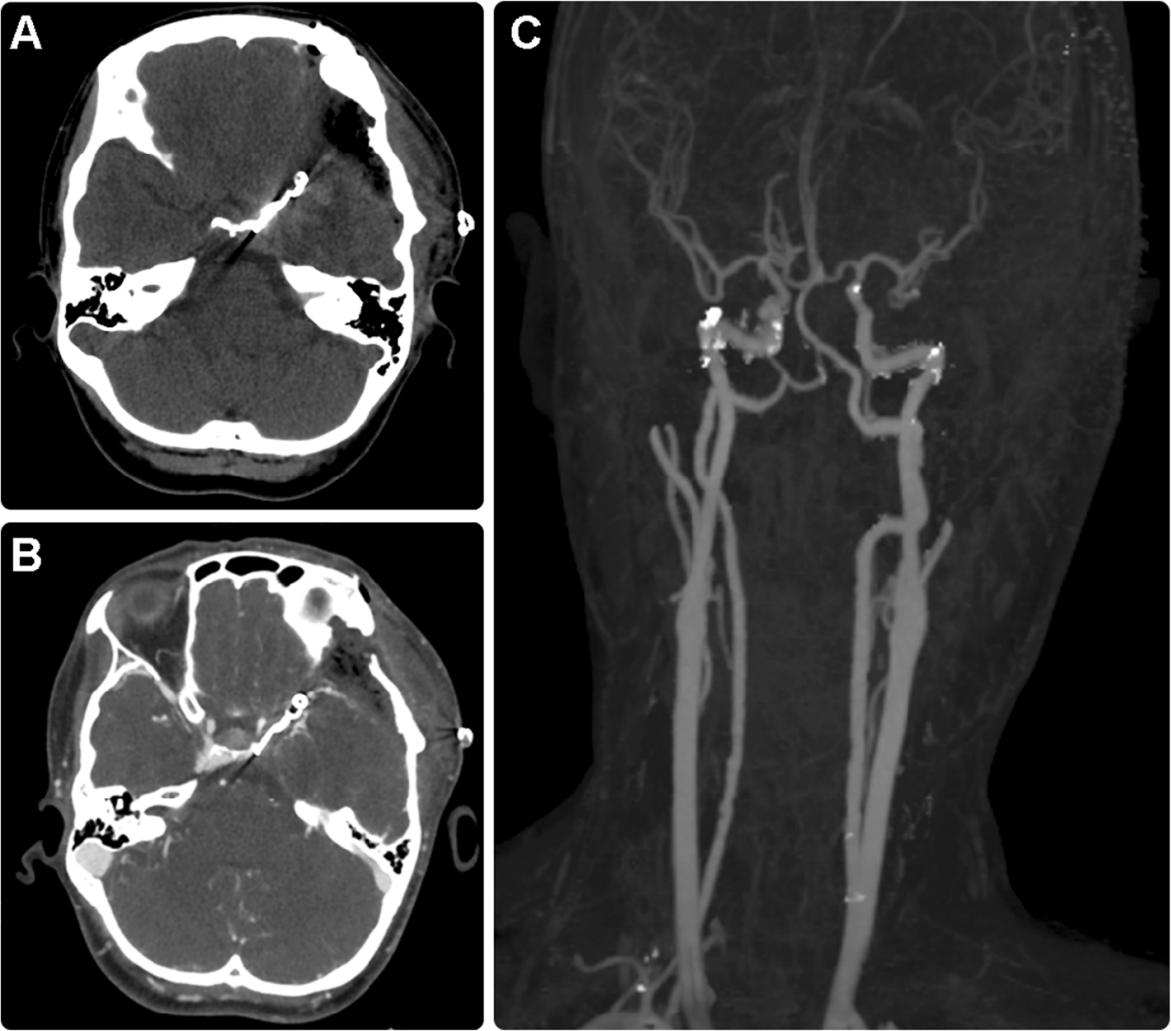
Axial unenhanced computed tomography (CT) scan of the brain (a), axial CT angiogram (b), and maximum intensity projection image (c) showing clipped matter in situ with no evidence of aneurysmal sac recanalization or recurrence. Left frontotemporal craniotomy is shown with the expected postoperative changes
